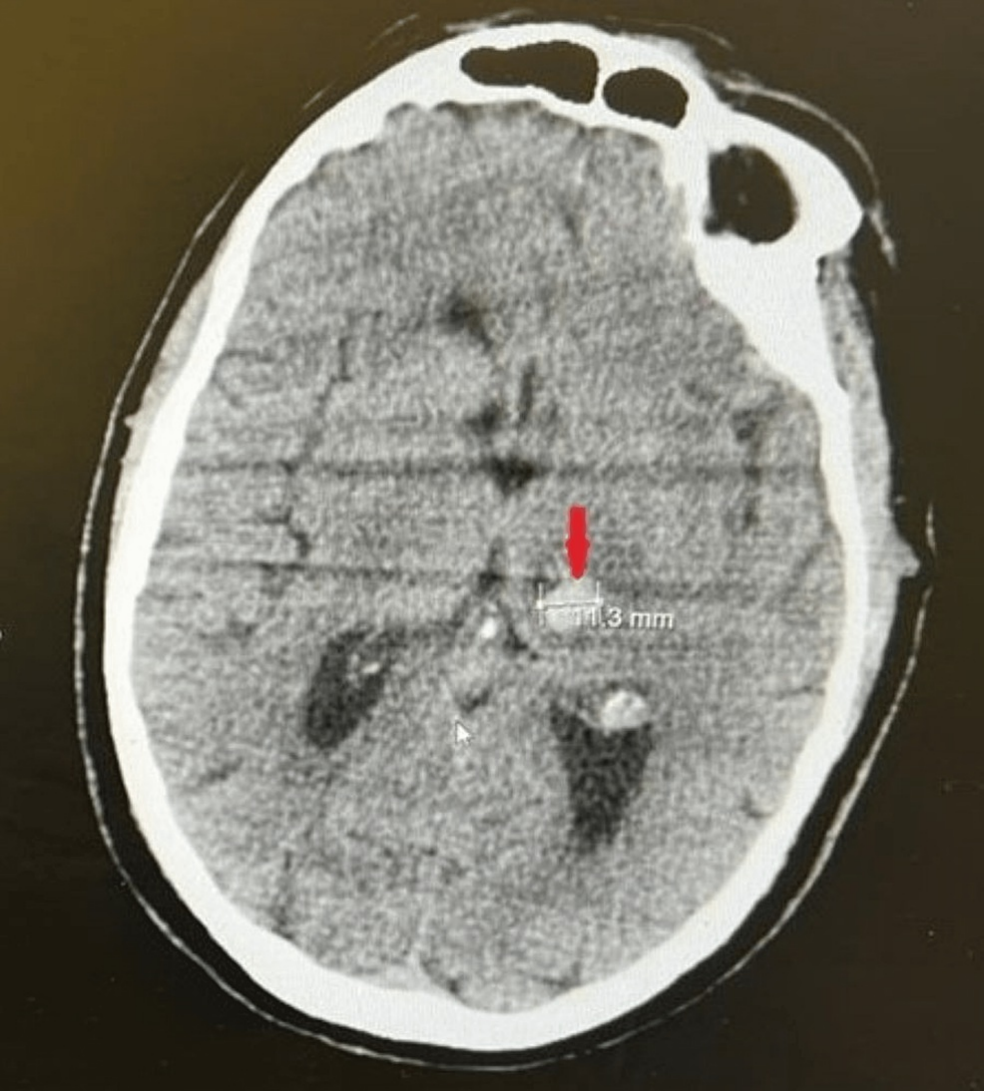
Axial image of a non-contrast CT of the brain post thrombolysis showing the same hyperdense left-sided intracranial hemorrhage (red arrowhead) measuring 11.3 mm with surrounding small volume edema indicating that there was no worsening or expansion of the intracranial bleed after alteplase administration.
